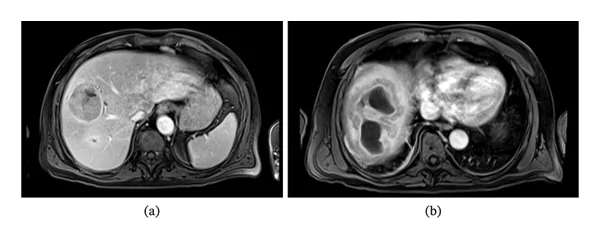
(a) Hepatic abscess containing a focal hepatocellular carcinoma component within segment V on T1‐weighted abdominal MRI. (b) Necrotic hepatic cavities involving segments VII and VIII on T1‐weighted abdominal MRI.
